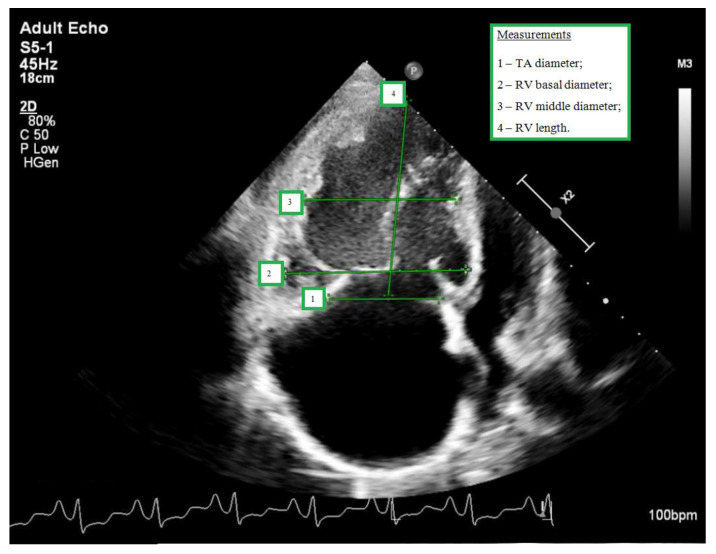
The 2D RV and TV echocardiographic measurements.
